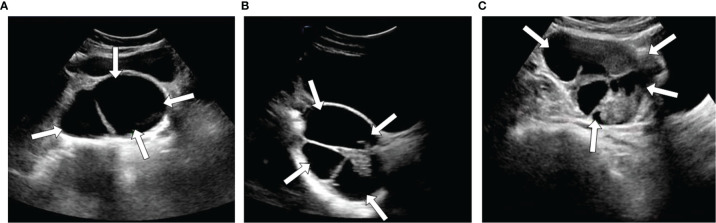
Three examples of ultrasound images with different types SOTs, benign (A), borderline (B), and malignant (C).
